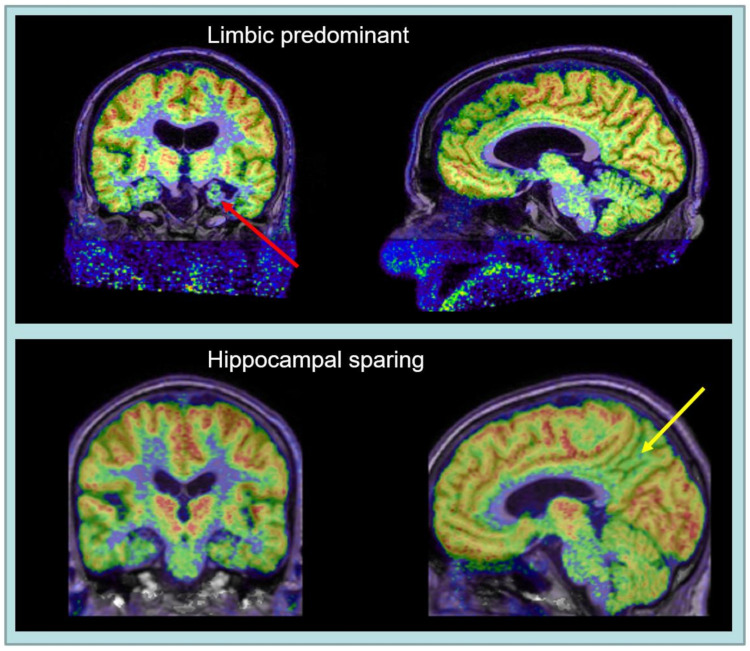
FDG PET with fusion overlay on T1-weighted MR, demostrating hippocampal hypometabolism and atrophy (red arrow) in limbic-predominant AD (top) and impaired parietal hypometabolism (yellow arrow) in a hippocampal-sparing AD (bottom). Both cases show metabolic impairment of posterior cingulate cortex.
